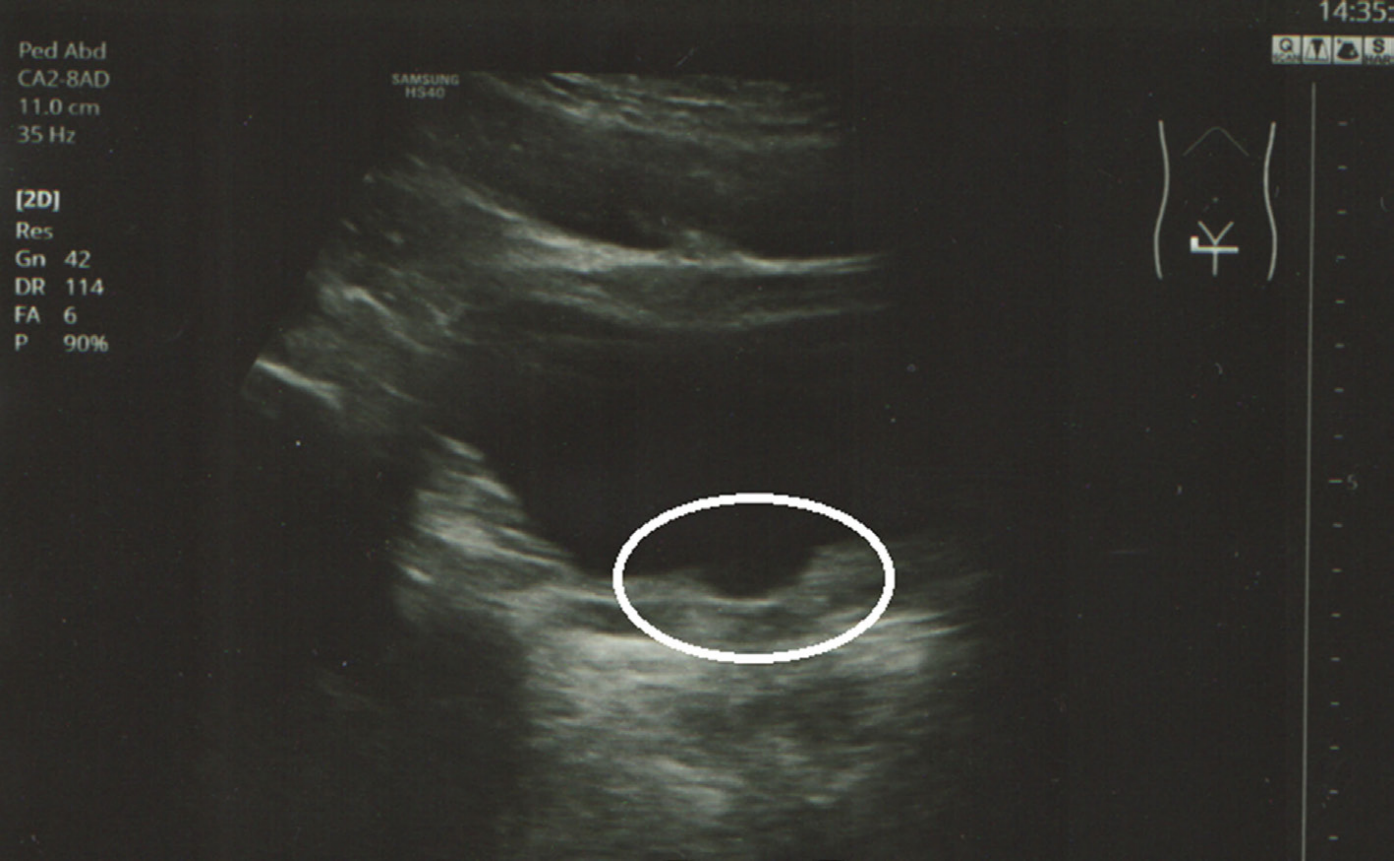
Transabdominal ultrasound: open bladder neck, horizontal view (circle).
